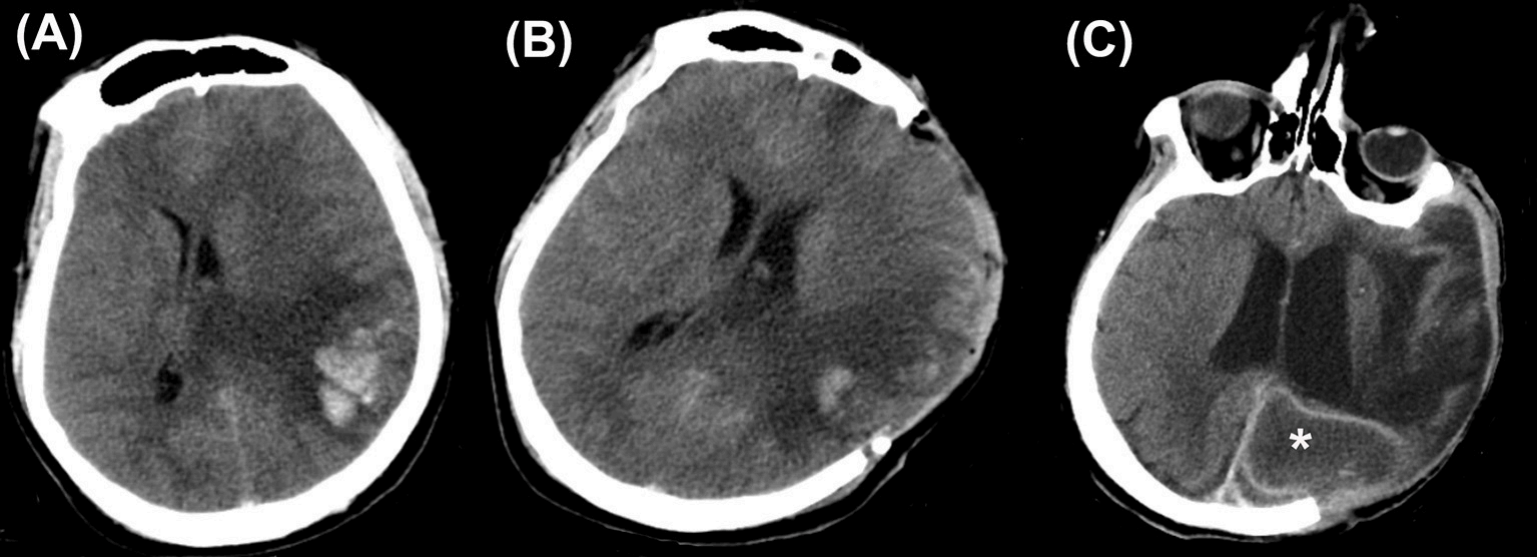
Infections. Computed tomography depicting (A) a case of cerebral venous sinus thrombosis. (B) Post craniectomy showed a reduction in the midline shift. (C) However, this patient developed brain abscess (asterisk) 2 months later.
